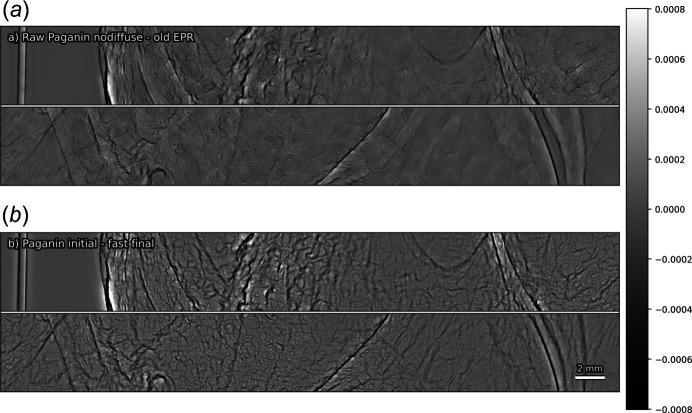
Direct comparison in the projection domain for a representative retrieved radiograph. Panel (*a*) shows the difference between a simple single-distance Paganin retrieval applied directly to the raw radiograph and the original EPR retrieved projection. Panel (*b*) shows the difference between the initial Paganin estimate used by the fast solver and the final fast retrieved projection. In each panel, the wide projection-difference image is split into left and right halves and these halves are stacked vertically for display, with a horizontal divider marking the join. Both panels are displayed with the same crop and the same grayscale range.
